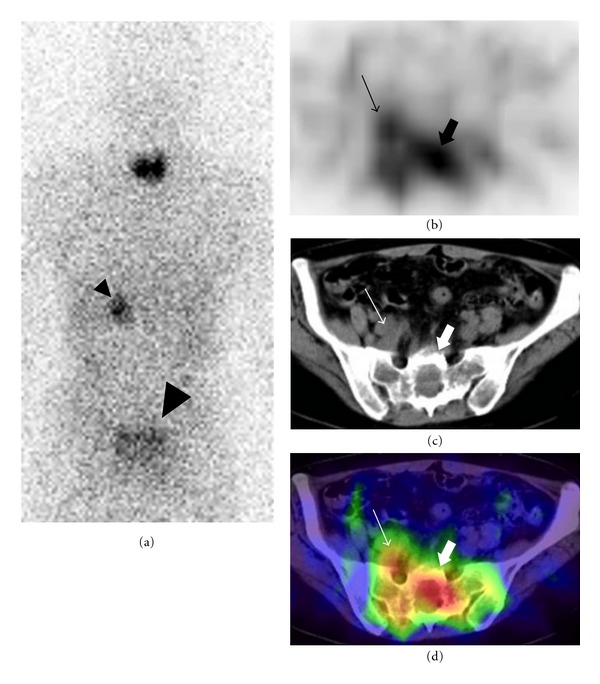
The posterior whole-body adrenocortical scintigraphy image showed focal uptake in the left adrenal (small arrowhead) and pelvic (large arrowhead) lesions (a). The axial SPECT (b), CT (c), and fused SPECT-CT (d) images confirmed increased uptake in the sacrum (thick arrows) and indicated increased uptake in the vein (thin arrows).
